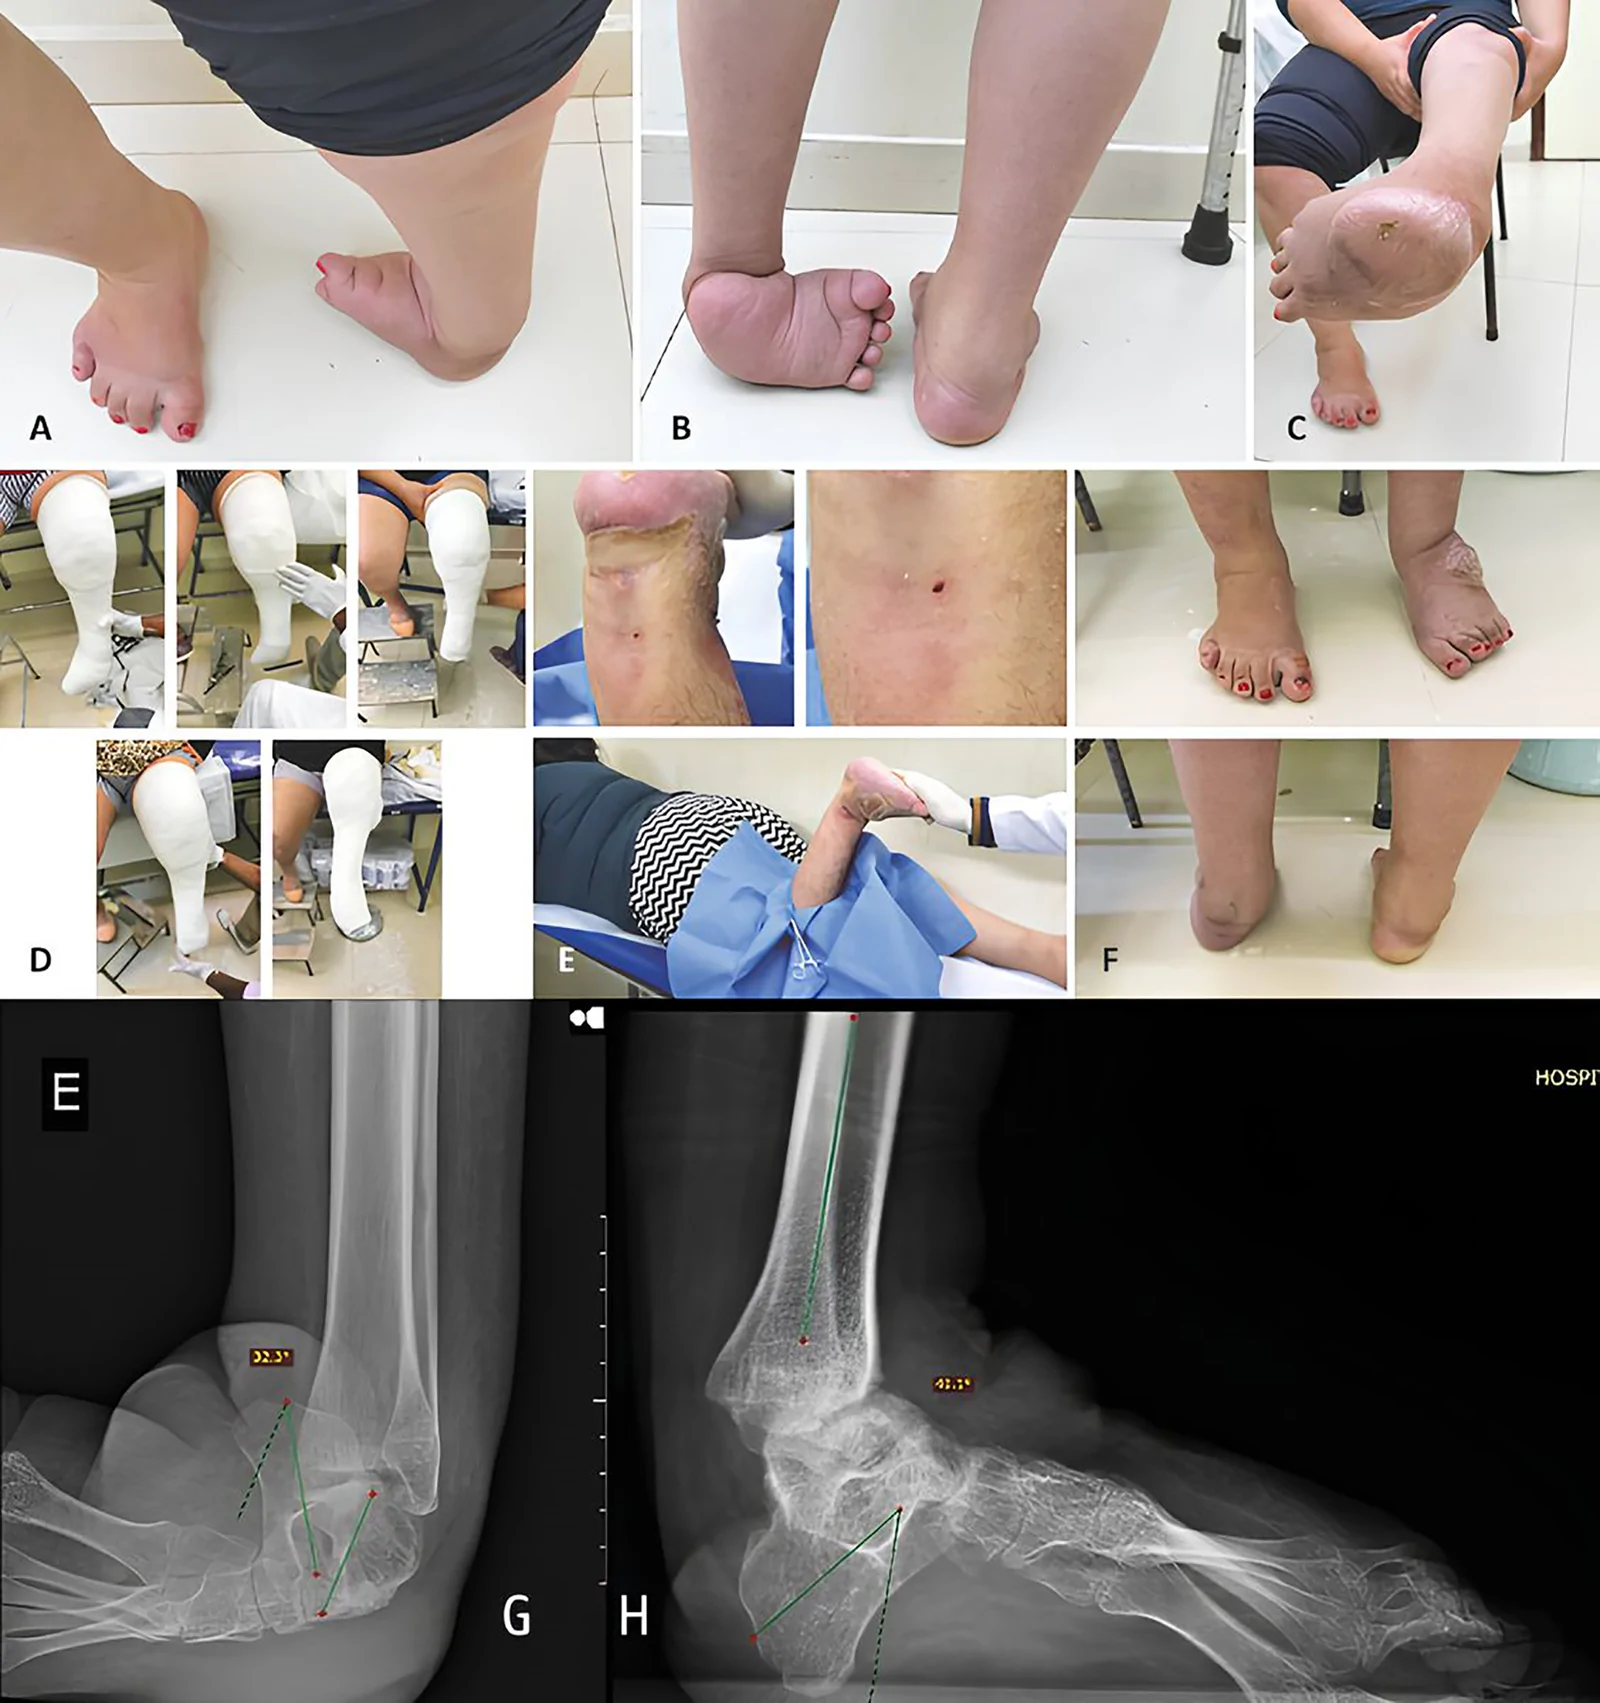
Clinical aspect of a rigid clubfoot of a myelomeningocele patient with obesity and recurrent ulcers before treatment (A, B, C). Sequential casts until 25° foot abduction (D). All the treatment was performed on an outpatient basis, even the Achilles tenotomy (with a minimum scar) and dorsiflexion maneuver to correct equinus (E). Final clinical aspect, with some degree of overcorrection (F). Radiographic appearance after treatment. Tibiocalcaneal angles are increasing due to equinus correction (G, H).
